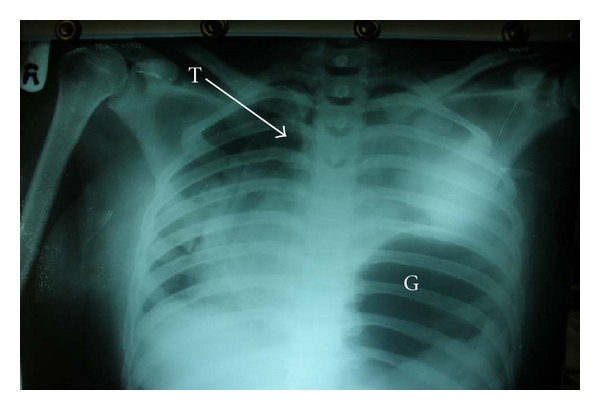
Preoperative mobile chest X-ray showing a large gastric shadow (G), absence of the left diaphragmatic outline and rightward displacement of the cardiac silhouette and trachea (T).
